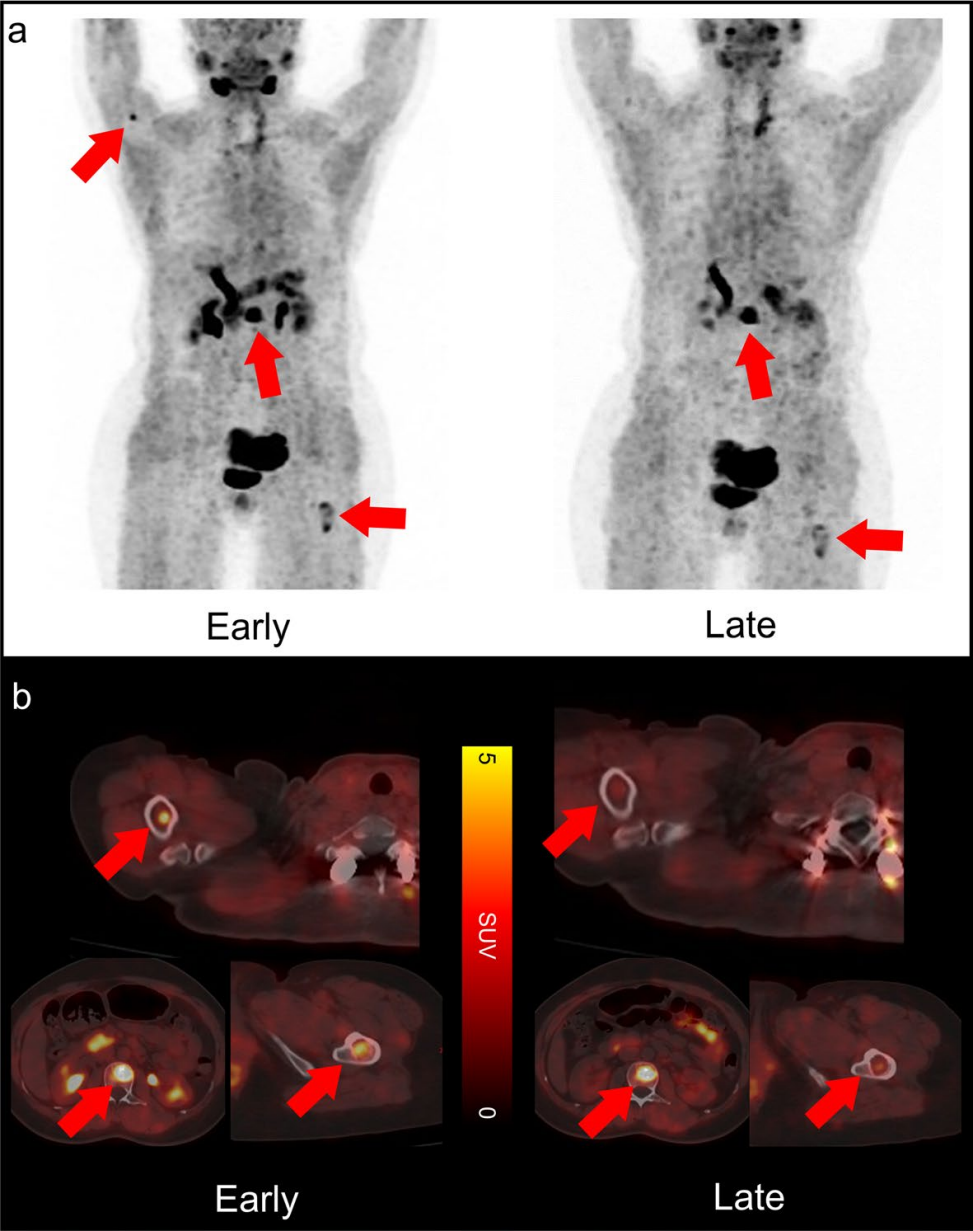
A 49-year-old woman with multiple bone metastasis of differentiated thyroid cancer. a Comparison of maximum-intensity projection images between early and late [^18^F]FAPI-42 PET (lesions indicated by arrows). b PET/CT images on early and late [^18^F]FAPI-42 PET (arrows indicate metastatic lesions). Early [^18^F]FAPI-42 PET detected more metastatic bone lesions than late [^18^F]FAPI-42 (SUV_max_ of humerus lesion is 4.9 and 2.4, respectively; SUV_max_ of vertebrae is 7.6 and 6.4, respectively; SUV_max_ of femur is 4.8 and 4.1)
